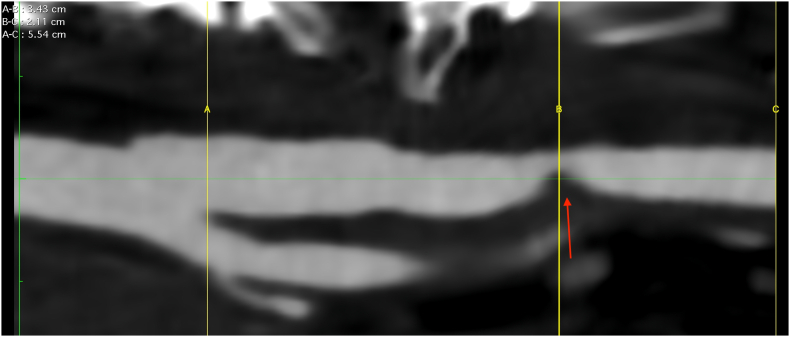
Preoperative CT Angiography centerline showing stenosis, distance from origin of internal carotid and length of plaque.
